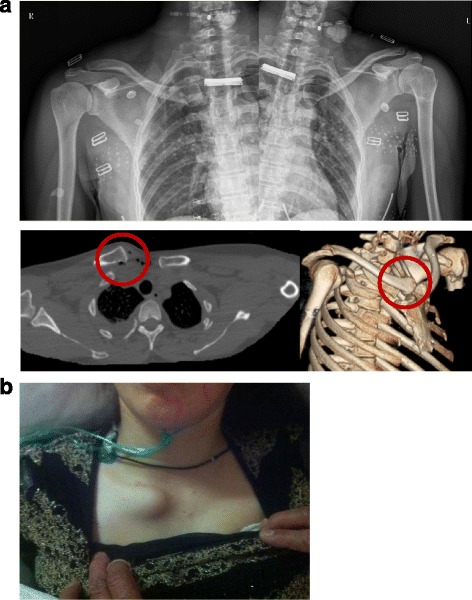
In patient 3, a 43-year-old female, the preoperative x-ray and computed tomography scan (a) show a right anterior dislocation of the sternoclavicular joint and a left proximal humeral fracture. A preoperative photograph (b) showing an obvious prominence at the medial end of the clavicle
